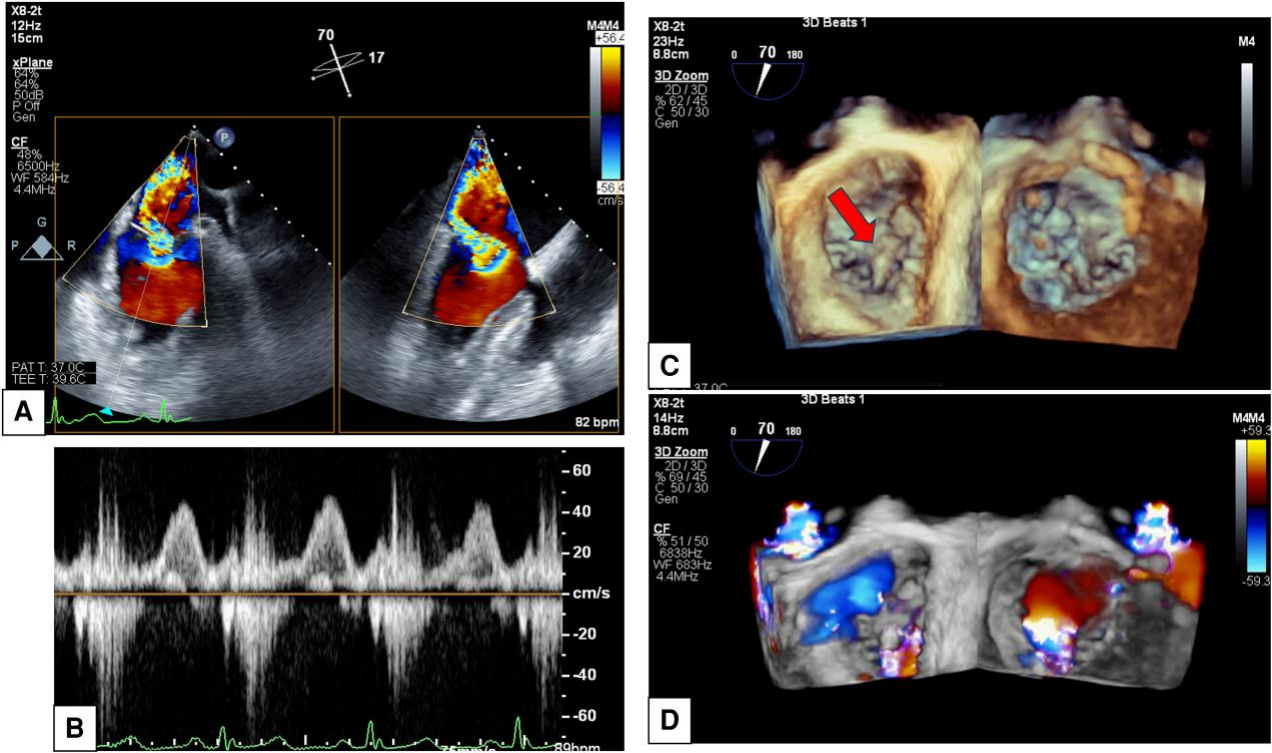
TEE findings. TEE reveals AML2 prolapse with ruptured chordae and severe regurgitation from the centre of the mitral valve. (*A*) Mitral commissural view and left ventricle and aorta long axis view. A deviated MR jet is observed originating from the prolapsed AML2. (*B*) Pulse wave Doppler findings in the left superior pulmonary vein. Pulmonary vein systolic flow reversal and finding of the S-wave being lower than the D-wave are observed. (*C*) Three-dimensional of mitral valve view from the left atrium and left ventricle. Prolapsed AML2 is observed (red arrow). (*D*) Three-dimensional of mitral valve view from the left atrium and left ventricle. Regurgitation flow from the prolapsed AML2 is confirmed, and flow convergence is observed on the left ventricular side.
